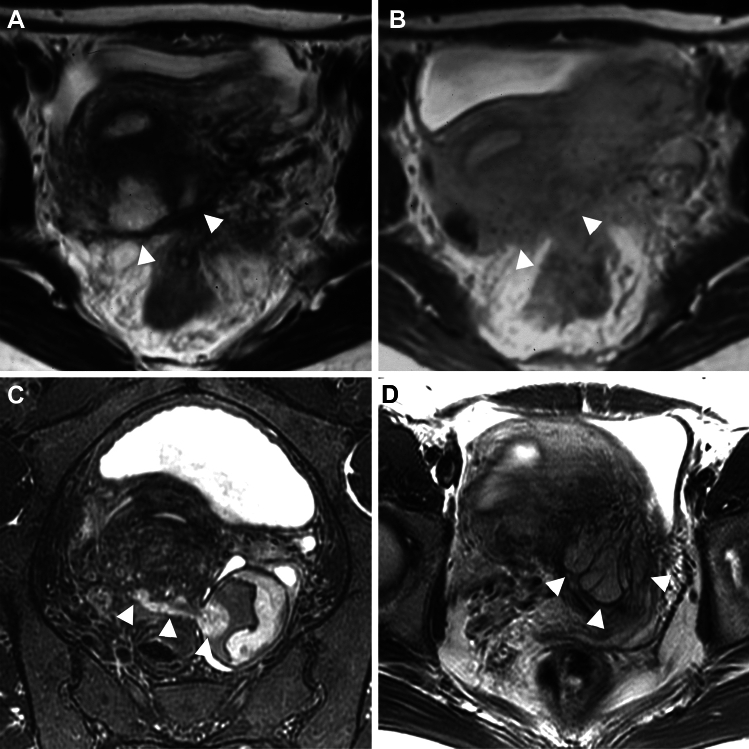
Polypoid endometriosis. A 47-year-old with lower abdominal pain associated with genital bleeding and suspicion of deep endometriosis. A T2-weighted image and B post-contrast T1-weighted image show polypoid masses (arrowheads) exhibiting T2-high signal intensity and intense contrast-enhancement protruding to the posterior wall of the uterine body with adenomyosis. The masses are surrounded by T2-low signal intensity adhesive fibrous tissue as “black rim sign”. The fibrous rim also shows intense contrast enhancement. A 33-year-old with a history of hypermenorrhea and dysmenorrhea and suspicion of deep endometriosis. C. Oblique coronal fat-saturated T2-weighted image shows a left endometrioma with high signal intensity mural nodule that extends to the Douglas' pouch (arrowheads). A 30-year-old with a history of dysmenorrhea and suspicion of deep endometriosis. D T2-weighted image shows polypoid masses infiltrating into the myometrium (arrowheads)
